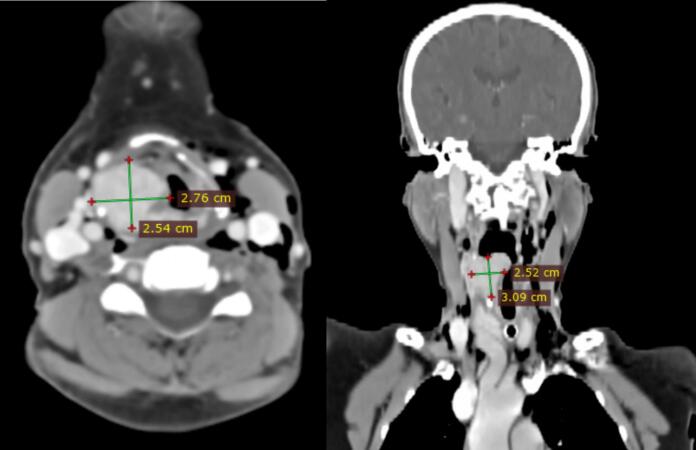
Head and neck CT scan with contrast (Fig. 2) showed a 2 × 3 cm enhancing mass in the right supraglottic region extending to the pyriform sinus.
